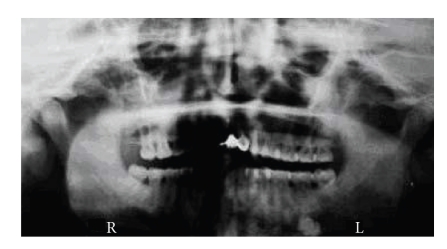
Panoramic radiograph shows an osteoma of the left angle of the mandible.
